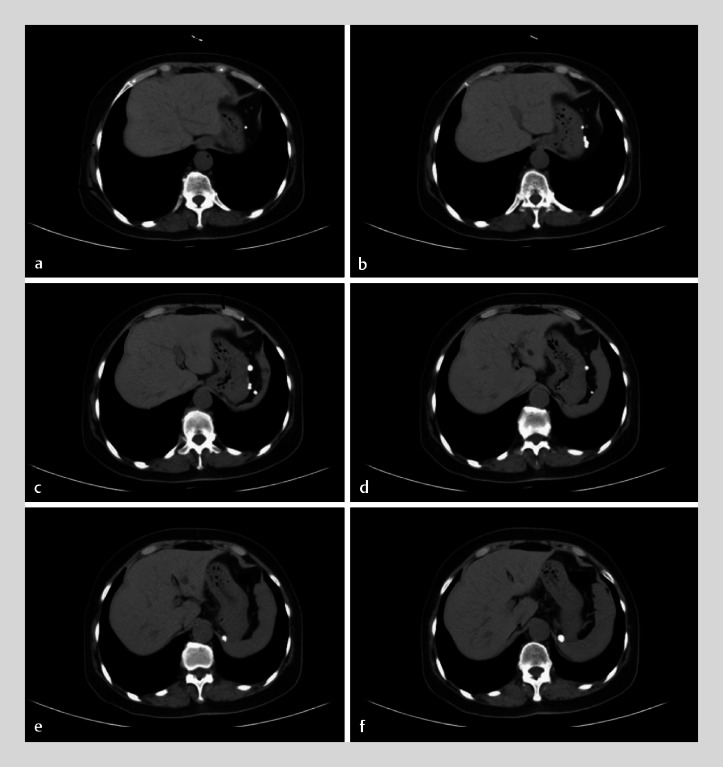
Computed tomography image series showing multiple, high-density, round lesions but no evident lesion on the gastric wall.
